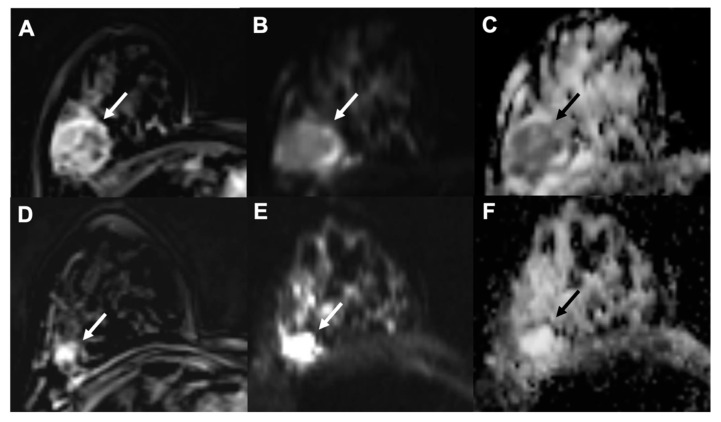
Example of early assessment of the response to NAC using diffusion weighted imaging (DWI). (A–C) = pre-NAC examinations; (D–F) = early assessment examination after two cycles of cytotoxic NAC. (A,D) = dynamic post-contrast images; (B,E) = DWI images; (C,F) = ADC maps. A 37-year-old patient with a G3, triple negative invasive ductal carcinoma of the right breast (white and black arrows). Early assessment showed a reduction of tumor size along with increase of signal intensity on ADC maps (C) compared to the pre-treatment examination (F). Pathology after surgical resection revealed pathological complete response (pCR).
